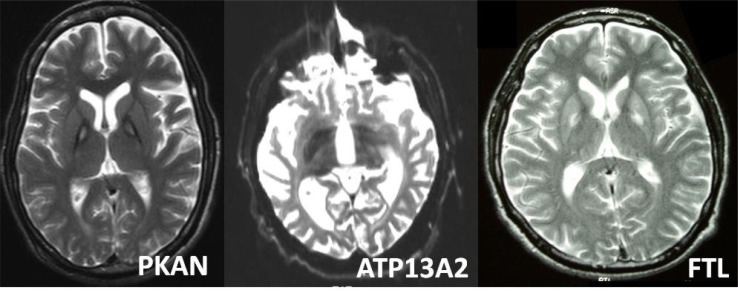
Examples of brain MR imaging in NBIA disorders; showing a case of pantothenate kinase associated neurodegeneration (PKAN)
